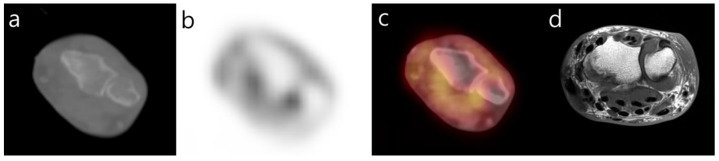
Axial ^18^F-FDG PET-CT image (a–c) of the left wrist demonstrates periarticular uptake and associated osseous erosions. Axial T1-weighted MR image (d) reconfirms erosion and periarticular hypointense amyloid deposits.
